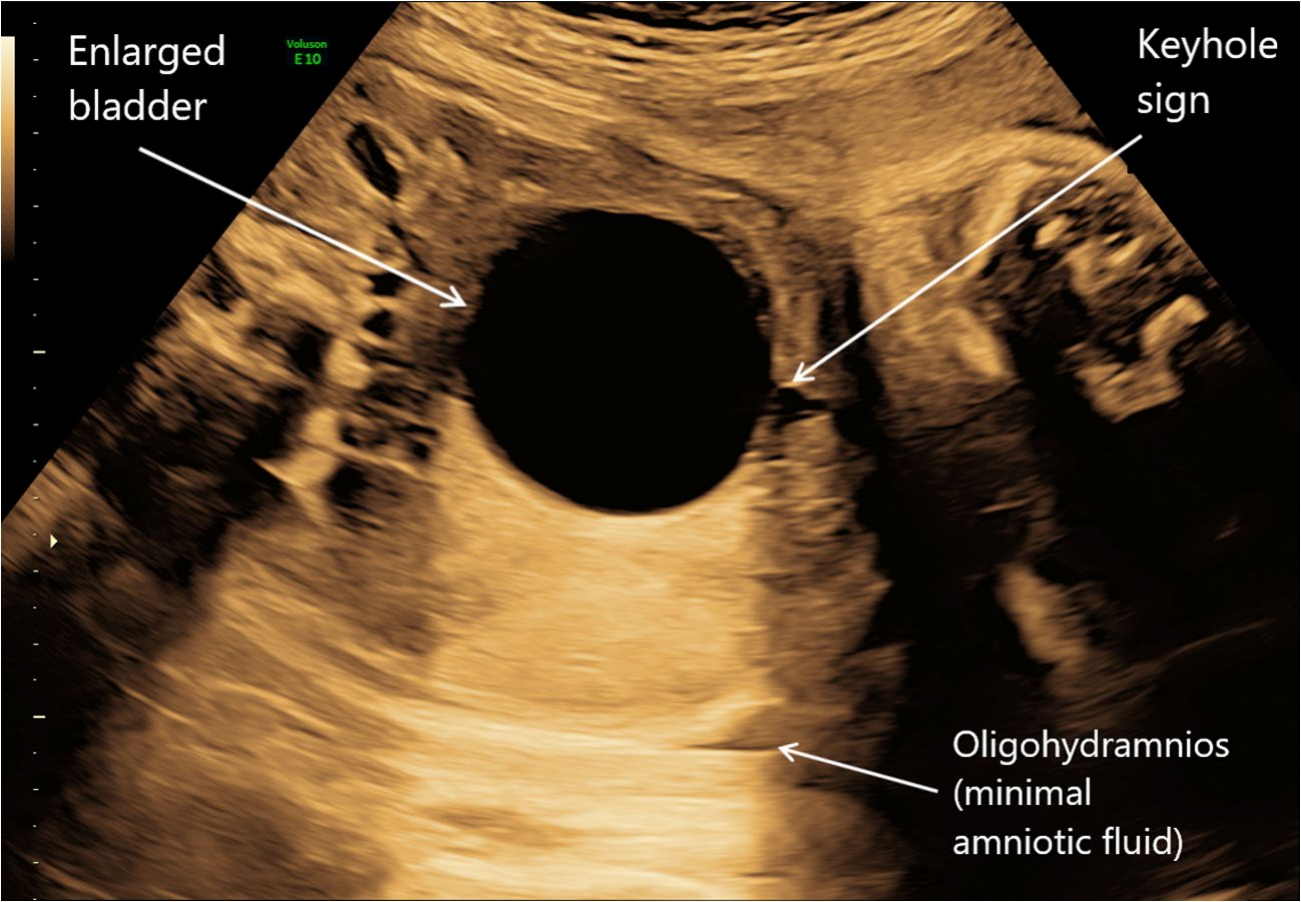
Antenatal ultrasound showing a distended bladder with ‘keyhole sign’ at 33/40 gestation with oligohydramnios. The infant was eventually confirmed as having posterior urethral valves postnatally
